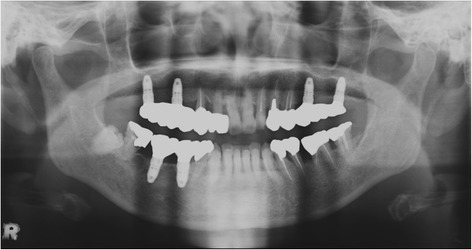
Postoperative panoramic radiograph taken about 3 years after implant loading
